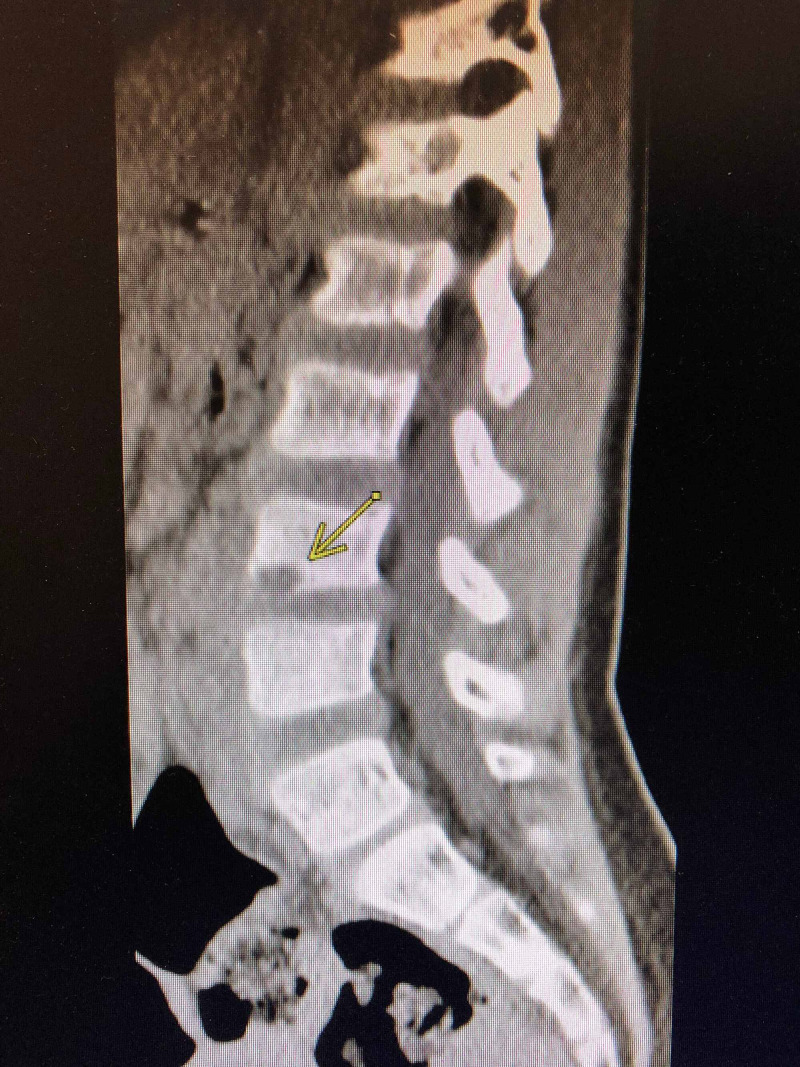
CT scan showing erosive changes in L4.
